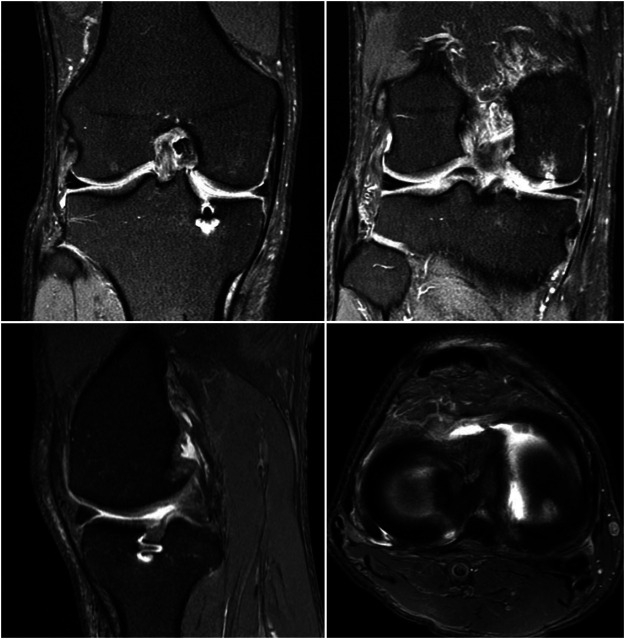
Coronal (A and B), sagittal (B), and axial (C) MR images of nonanatomic root repair patient #3 showing evidence of osteoarthritis in the medial compartment of the knee secondary to nonanatomic medial meniscus root repair (right knee). M = medial; L = lateral; A = anterior; P = Posterior.
